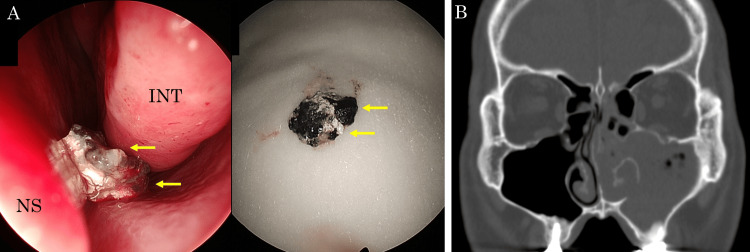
Intraoperative images and sinus CT after surgery (A) Rubber-like hard heterogeneous material flowed out from the maxillary sinus (arrows). (B) Sinus CT on the first postoperative day revealed no radiopaque mass. CT: computed tomography; INT: inferior nasal turbinate; NS: nasal septum
